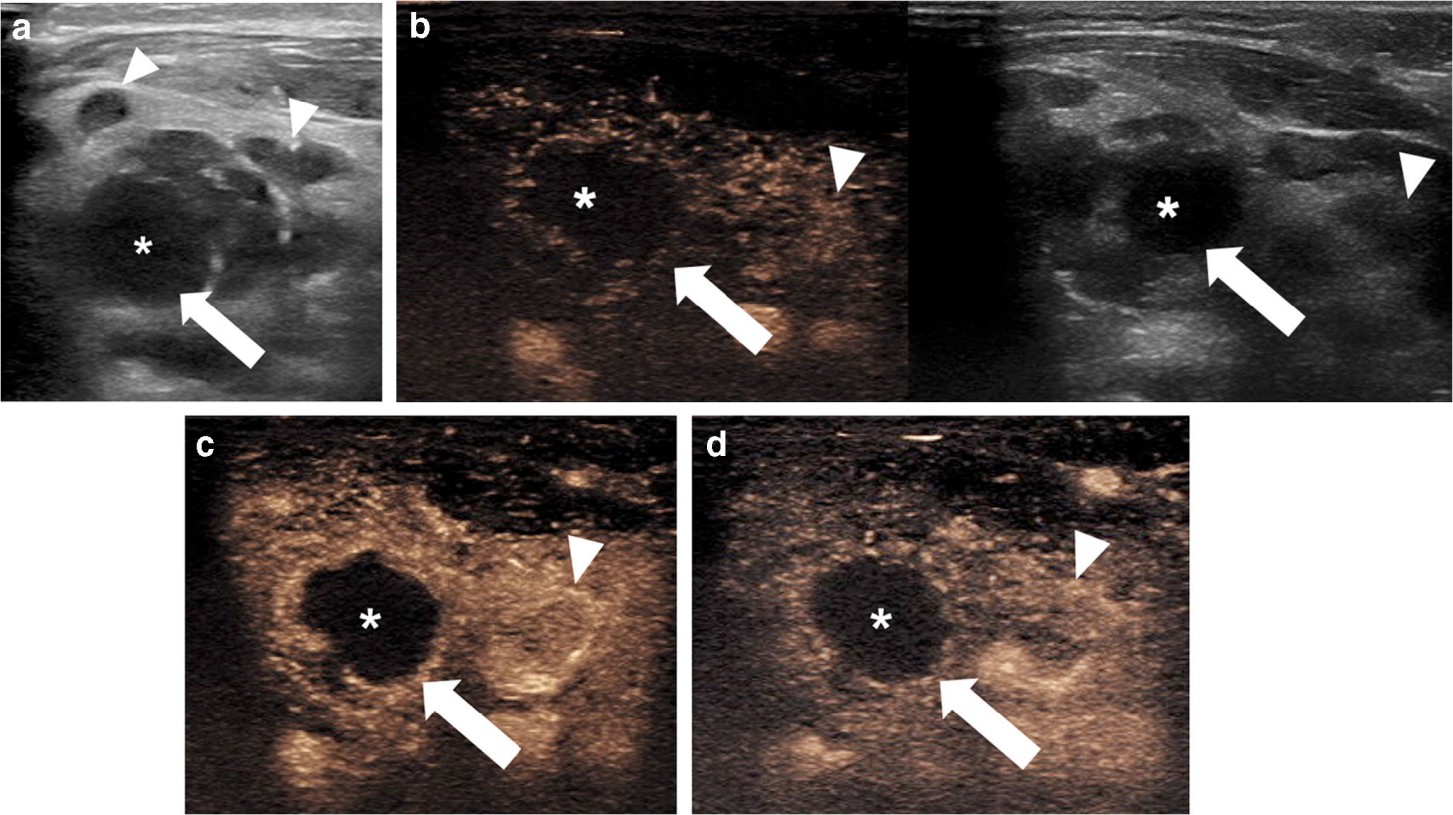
Severe right-side lymphadenitis colli in a 5-year-old girl. a Gray-scale US, transverse plane, shows multiple lymph nodes (*arrowheads*), one of which appears enlarged (*arrow*) with an anechoic area centrally (*asterisk*), suggestive of abscess formation. b–d Transverse contrast-enhanced ultrasound (CEUS) image with dual display of contrast (*left*) and gray-scale (*right*) modes and in contrast-only mode at 10 s (b), 15 s (c) and 39 s (d) post contrast injection. The central anechoic area of the lymph node (*asterisk*) demonstrates complete lack of enhancement corresponding to the purulent content. A hyperenhancing rim (*arrow*) is noted in the periphery corresponding to the adjacent inflamed tissue. An additional enlarged and abnormally enhancing lymph node is noted adjacent to the abscess (*arrowhead*)
